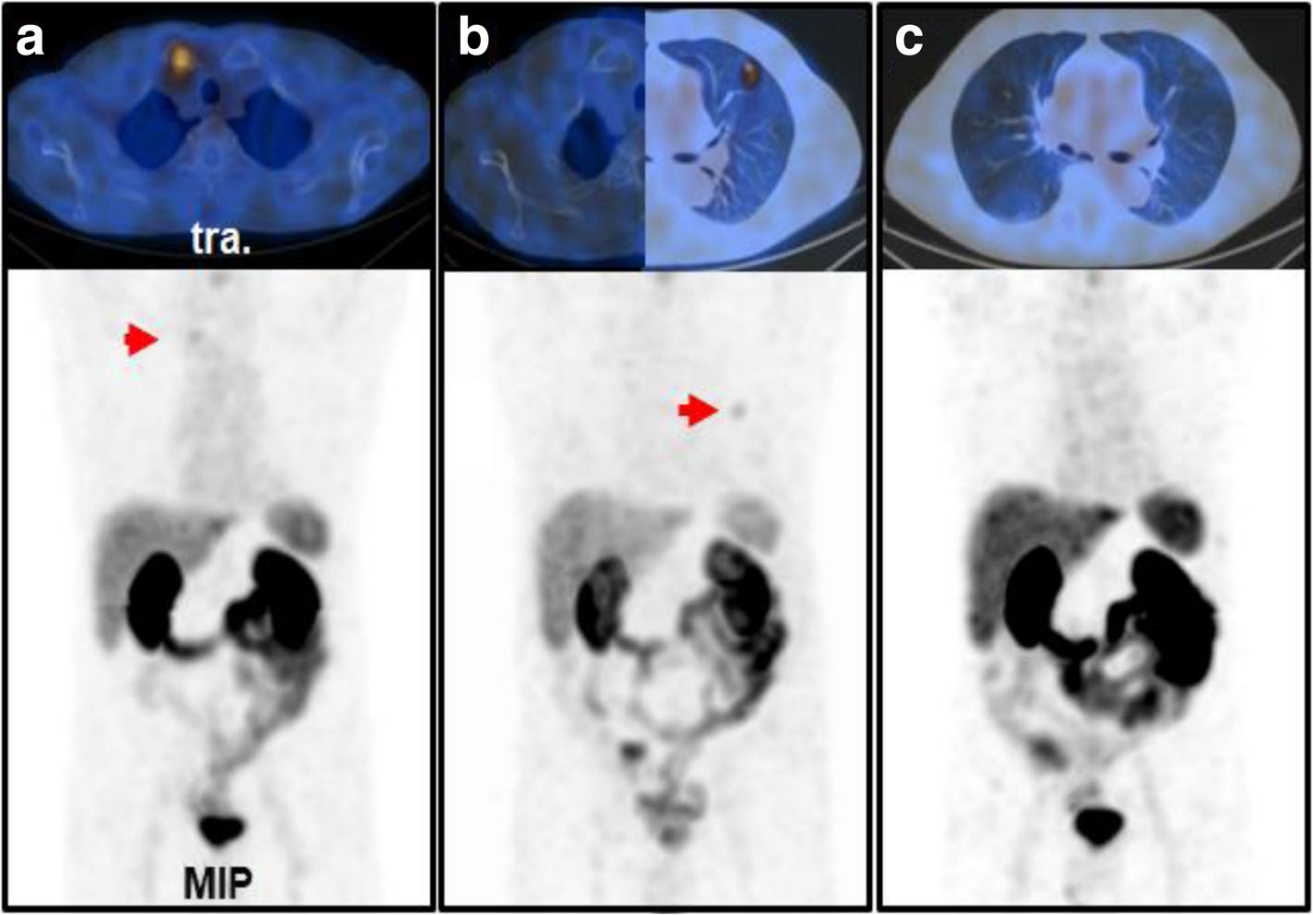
Lesion detection in BCR and monitoring of radiation therapy in a 72-year-old patient with Gleason 7 PCa, who underwent primary surgery and salvage radiation therapy. a Cancer recurrence was detected in the right clavicle (PSA 3.8 ng/ml) which was followed by targeted radiation. b 6 months later the clavicle was unremarkable but another recurrence was detected in the lung (PSA 6.8 ng/ml) again followed by precision radiotherapy. c Another 6 months later, no tumor recurrence was detected (PSA 0.56 ng/ml). Note: the apparent focal PSMA-positivity in the liver (c) could not be confirmed in the cross-sectional planes and was not categorized as PSMA-positive
